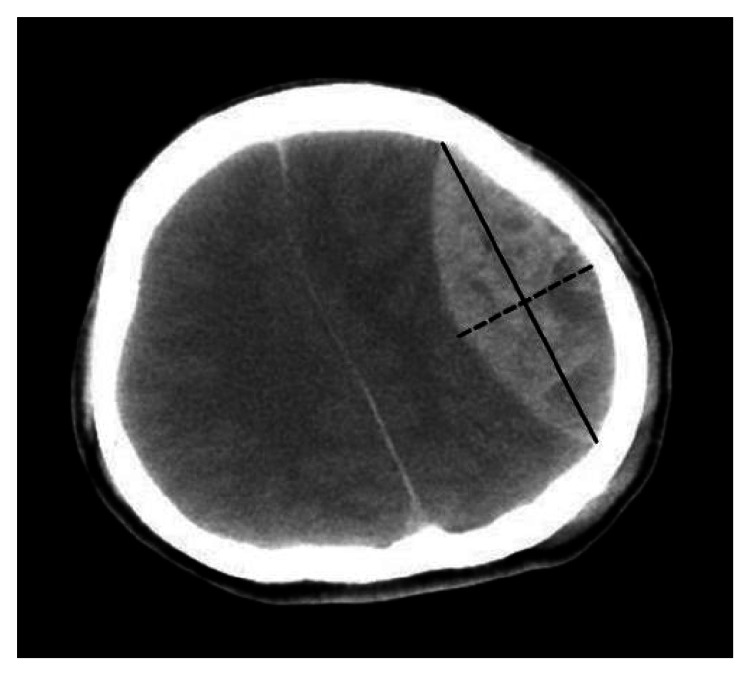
An epidural hematoma on an axial CT image showing its biconvex shape and bilateral symmetry along the long axis (solid line), as well as approximate bilateral symmetry along the short axis (dashed line).
